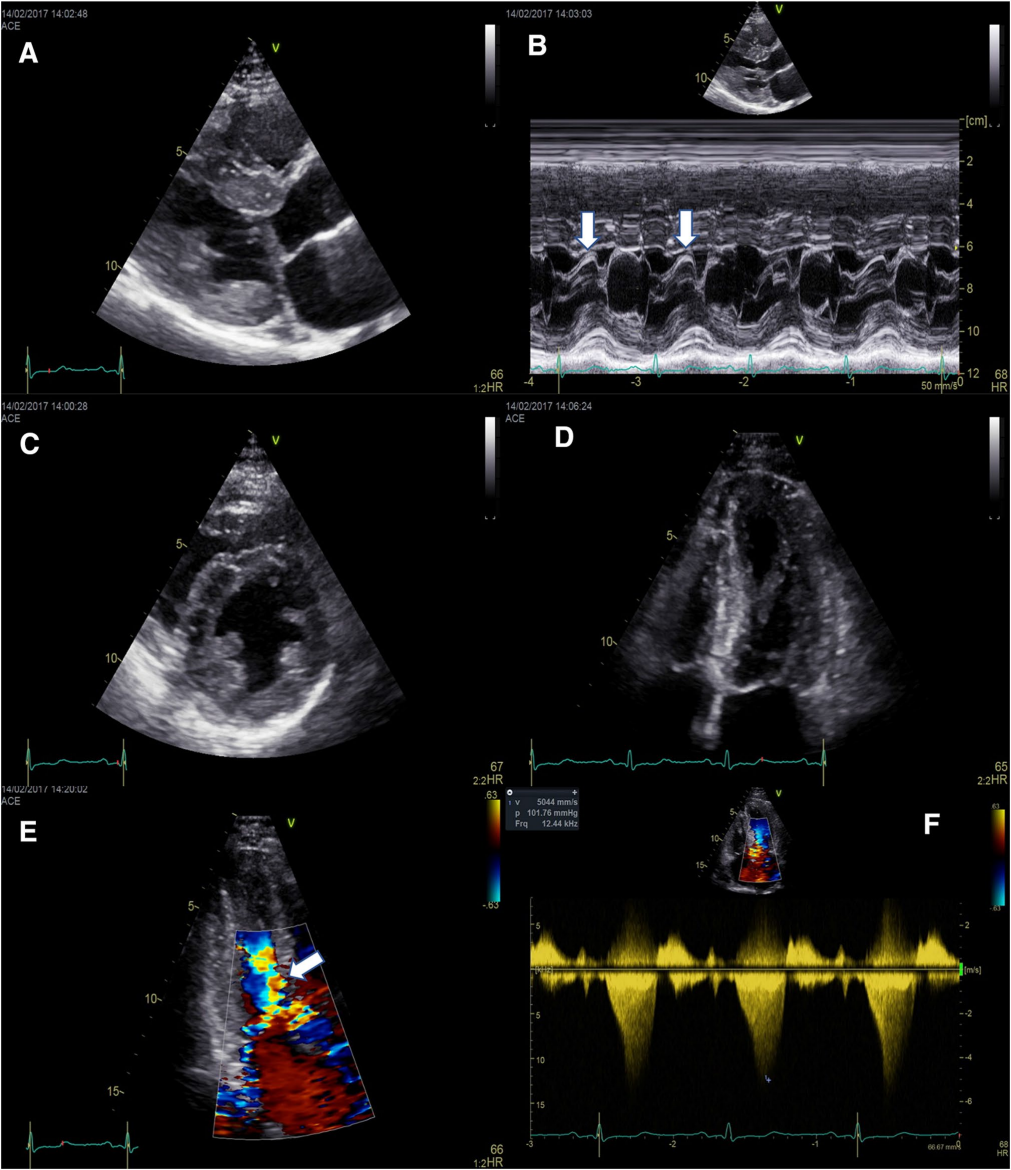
Complex mechanisms leading to dynamic obstruction in a patient with HCM. Concentric hypertrophy involving mainly the basal septum (diastolic IVS thickness of 15 mm), and elongated mitral leaflets with systolic anterior motion (a); M-mode echocardiography shows the systolic contact of the mitral valve with the IVS (arrows) (b); anterior displacement of the hypertrophied papillary muscles (c, d); moderate eccentric (posteriorly oriented) mitral regurgitation secondary to SAM (e); and significant resting LVOT obstruction by CW Doppler (peak resting gradient of 102 mmHg) (f). Of note, there is severe LVOT obstruction without severe septal hypertrophy, explained by the significant abnormalities of the mitral valve apparatus. *HCM* hypertrophic cardiomyopathy, *IVS* interventricular septum, *LVOT* left ventricular outflow tract
